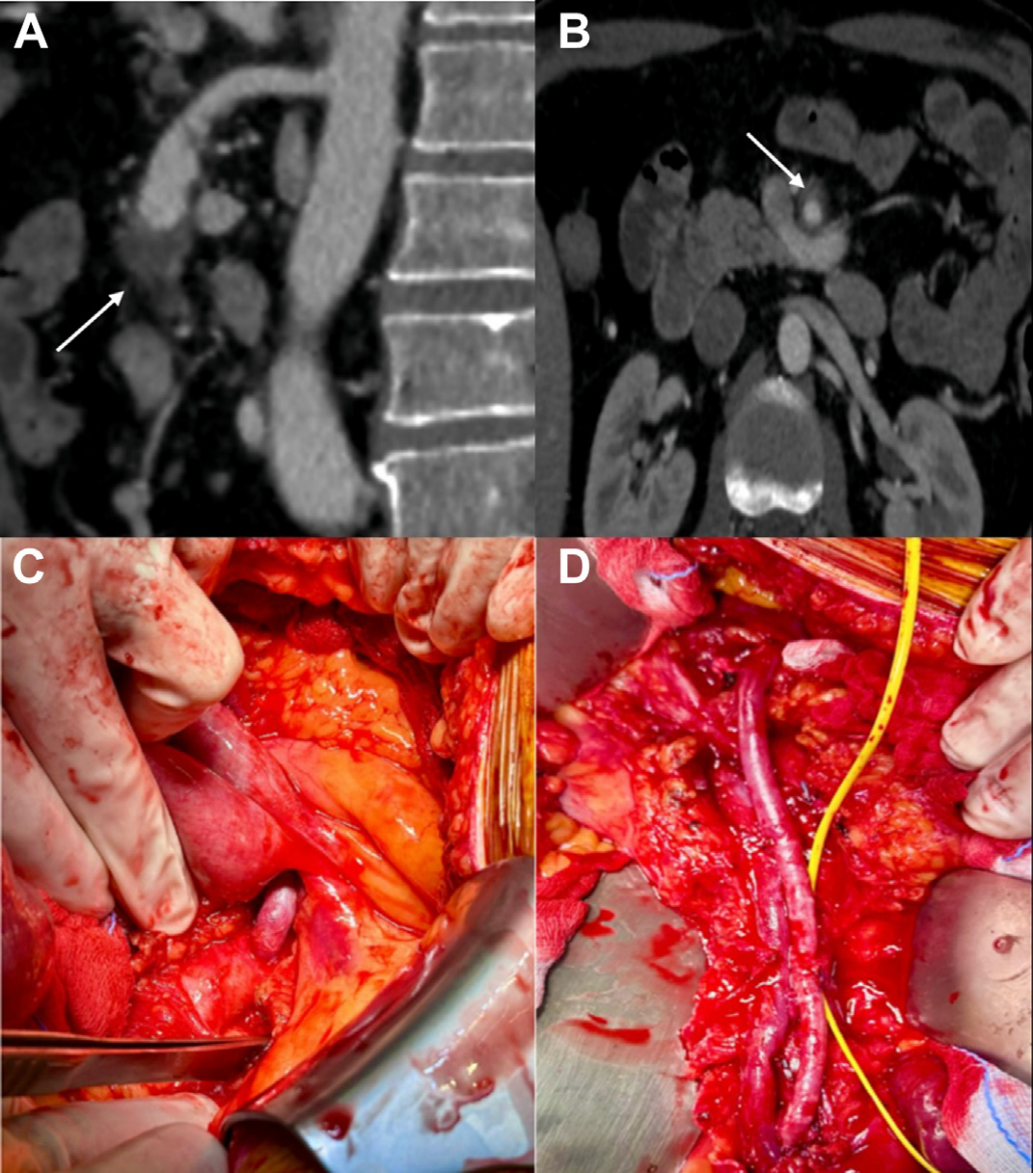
Case 1 – Preoperative Imaging and Surgical Outcome of Aortomesenteric Bypass for Thrombosed SMA Aneurysm Preoperative computed tomography angiography in sagittal (A) and coronal (B) views showing a thrombosed superior mesenteric artery aneurysm. (C) Intraoperative images depicting the proximal anastomosis between the infrarenal aorta and the great saphenous vein graft. (D) Final result: aortomesenteric bypass with distal anastomosis between the great saphenous vein graft and the superior mesenteric artery, along with reimplantation of the first ileocolic branch.
